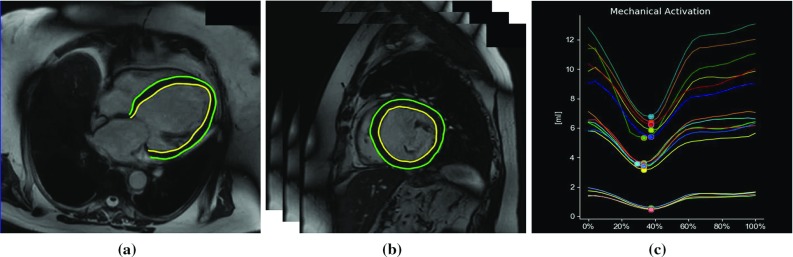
Pre-procedural a LA and b SA cine MRI acquisition, along with automatic outline of endocardial (yellow) and epicardial (green) contours in the acquired SA and LA images. c Display of regional volume curves expressed as a percentage of the total cardiac cycle for each of the 16 segments of the American Heart Association LV model
